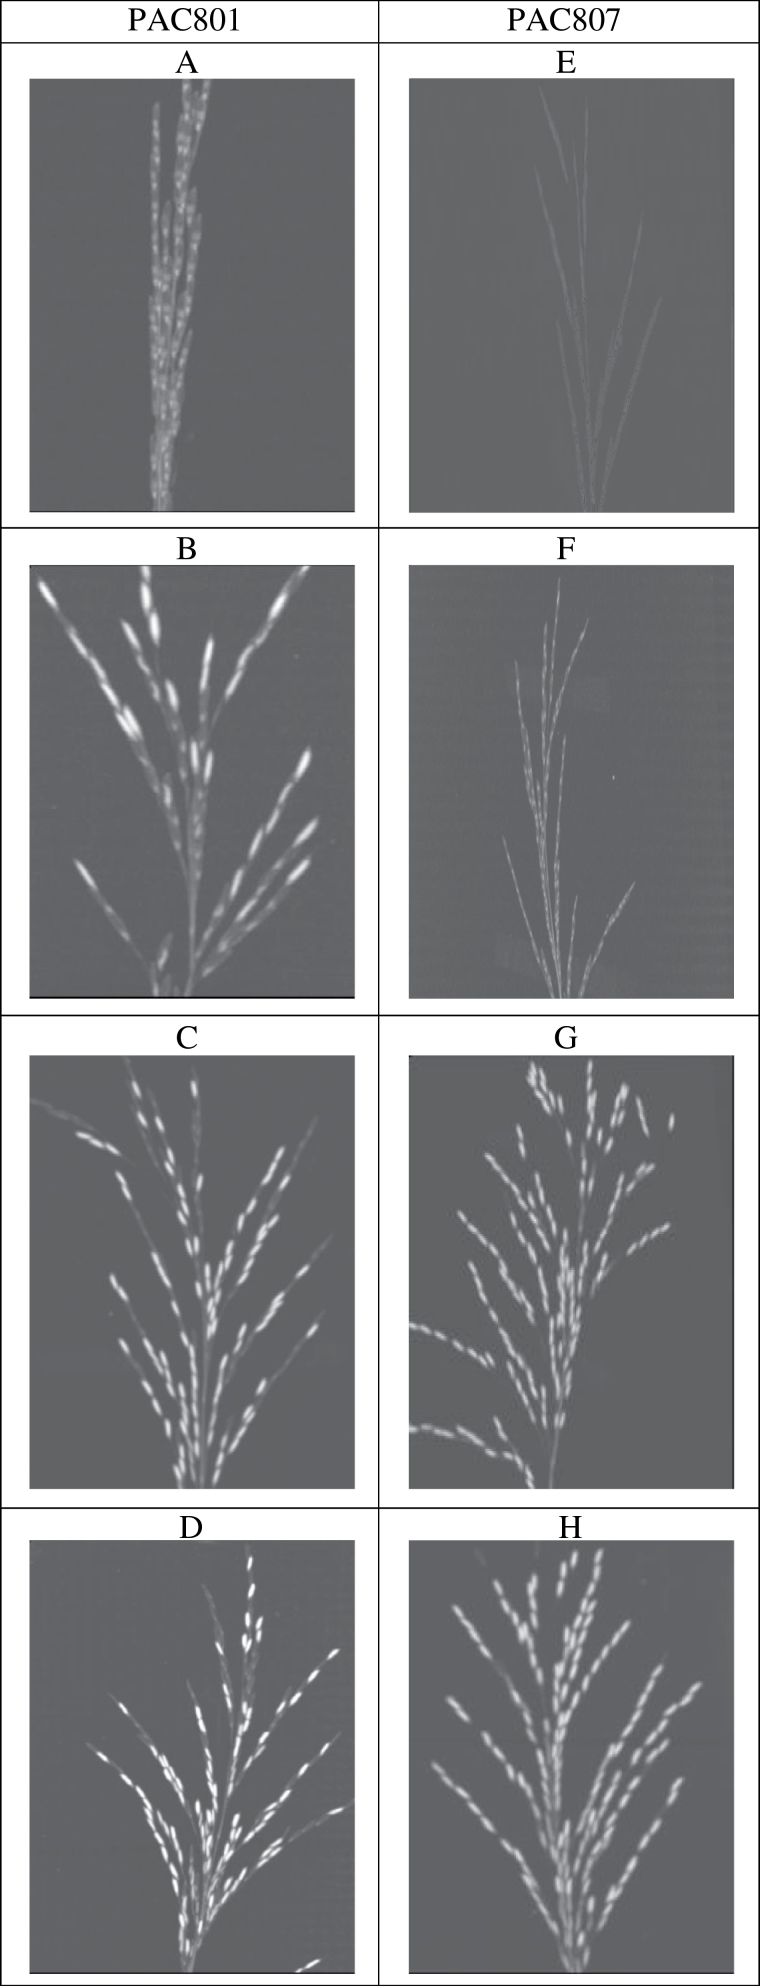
Spikelet images of rice varieties PAC801 (A–D) and PAC807 (E–H), at the initiation (A, E), elongation (B, F), dry matter accumulation (C, G), and maturation (D, H) phases, respectively. CT scan image is in the Multi-Planar Reformatting (MRP) tool to visualize images for calculating various topological parameters (for other details see Supplementary Fig. S1 at *JXB* online).
